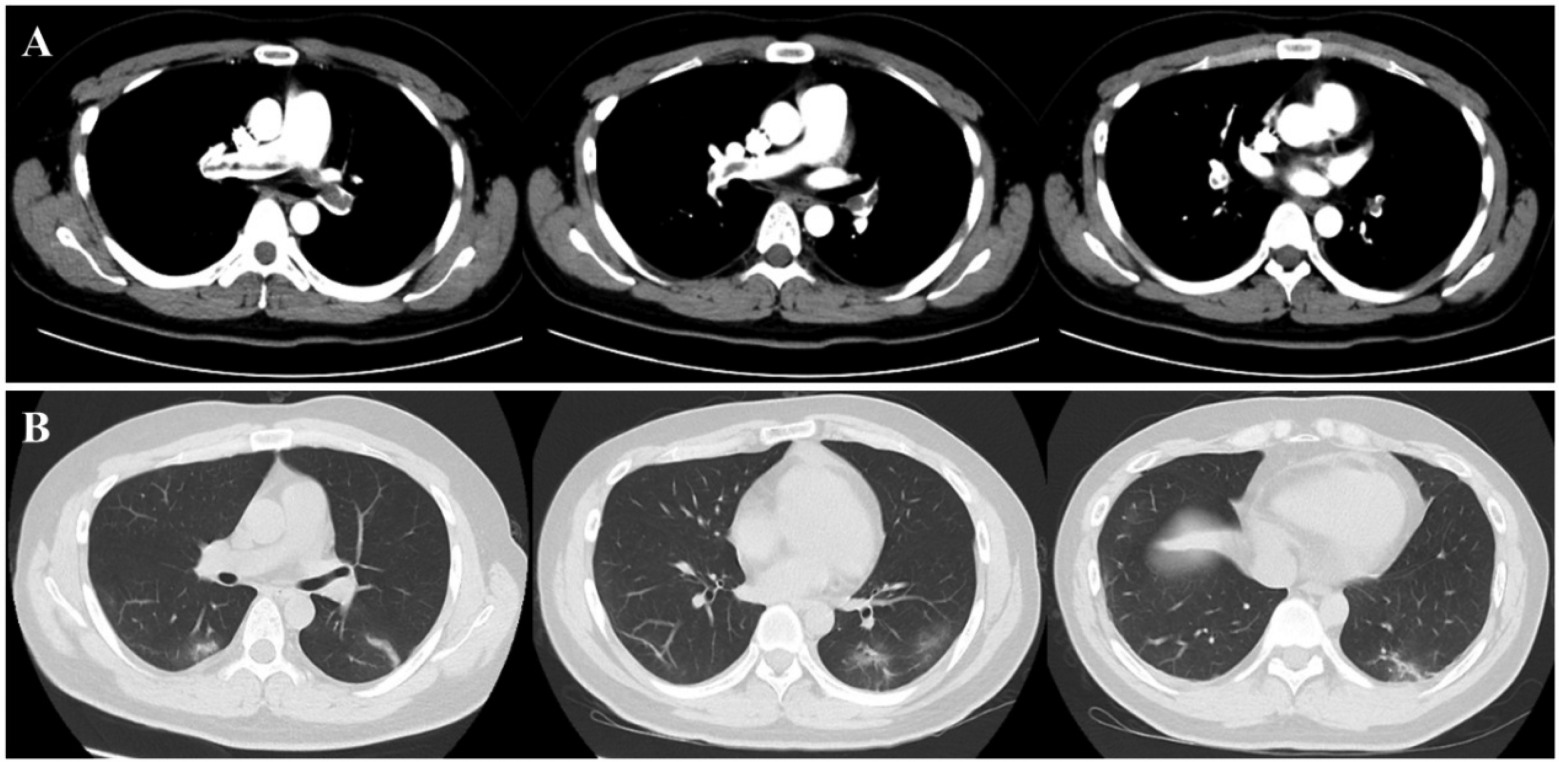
Present of thrombus in pulmonary veins and inflammatory changes in lung. The chest CT scan revealed the presence of multiple emboli in the pulmonary artery (A), as well as inflammation (B) in the lower lobes of both lungs.
